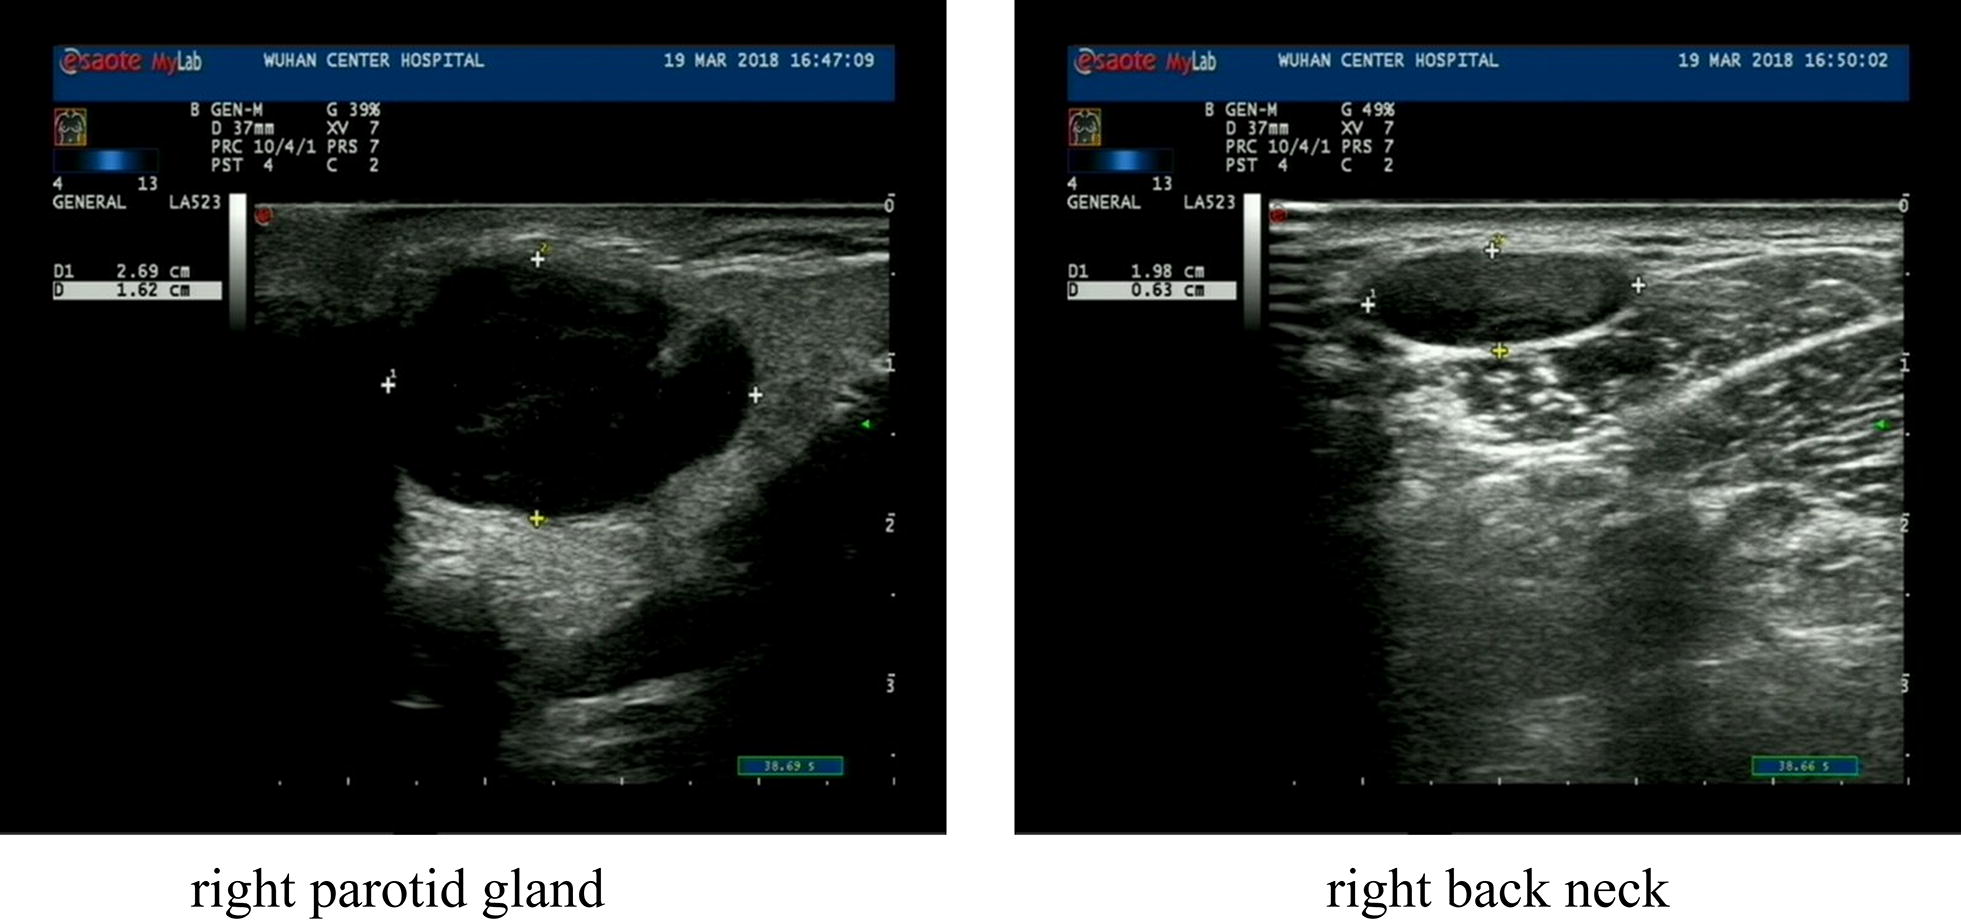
Ultrasonography showing enlarged lymph nodes in right parotid gland and back neck
